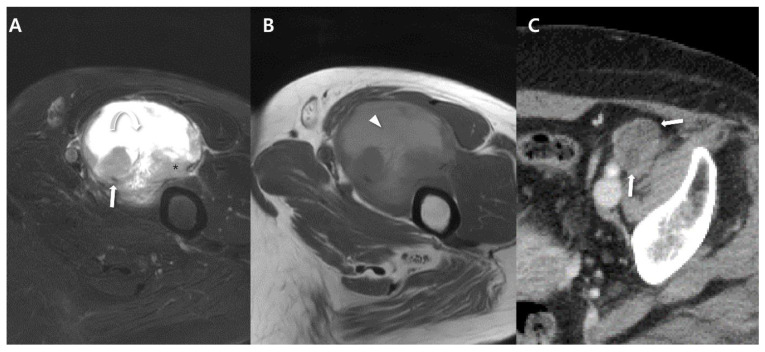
Lymph node metastasis from synovial sarcoma (same patient with Figure 3): (A,B) Initial MRI of axial T2-weighed, fat-suppressed and T1-weighted images shows about 6.3 cm sized soft-tissue mass with triple sign (curved arrow: hyperintense, *: isointense, arrow: hypointense) and hemorrhagic change (arrowhead). (C) Follow-up CT after 1 year shows regional lymph node metastasis (arrows).
